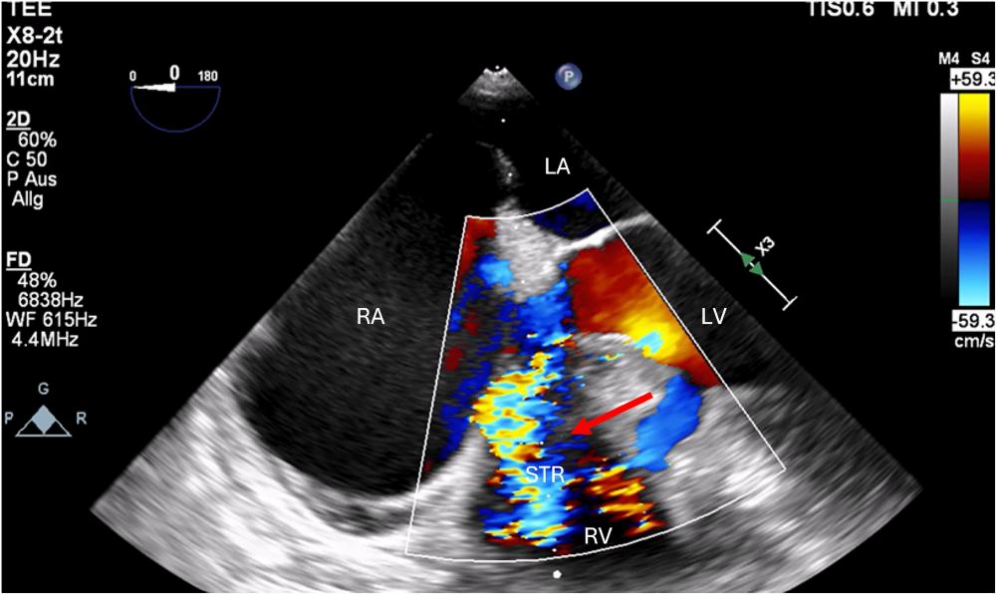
Transoesophageal echocardiography. Severe tricuspid regurgitation (marked by red arrow) with dilation of the right heart chambers; RA, right atrium; LA, left atrium; LV, left ventricle; RV, right ventricle; STI, severe tricuspid regurgitation (©Medical University of Graz).
